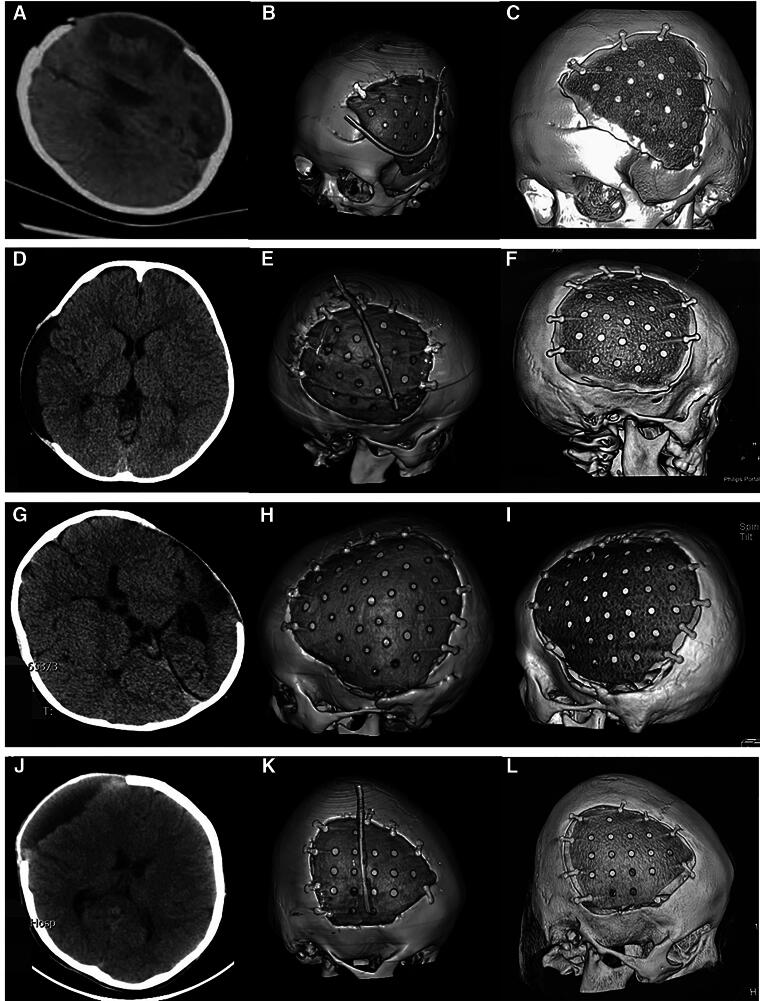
CT images of four typical patients with bone gap expansion at the interface between cranial bone and PEEK. (A, D, G, J) Preoperative CT image. (B, E, H, K) Postoperative 3D CT image. (C, F, I, L) Follow-up 3D CT image. Patient 1: A, B, C; patient 2: D, E, F; patient 3: G, H, I; patient 4: J, K, L. CT, computed tomography; PEEK, polyether ether ketone.
